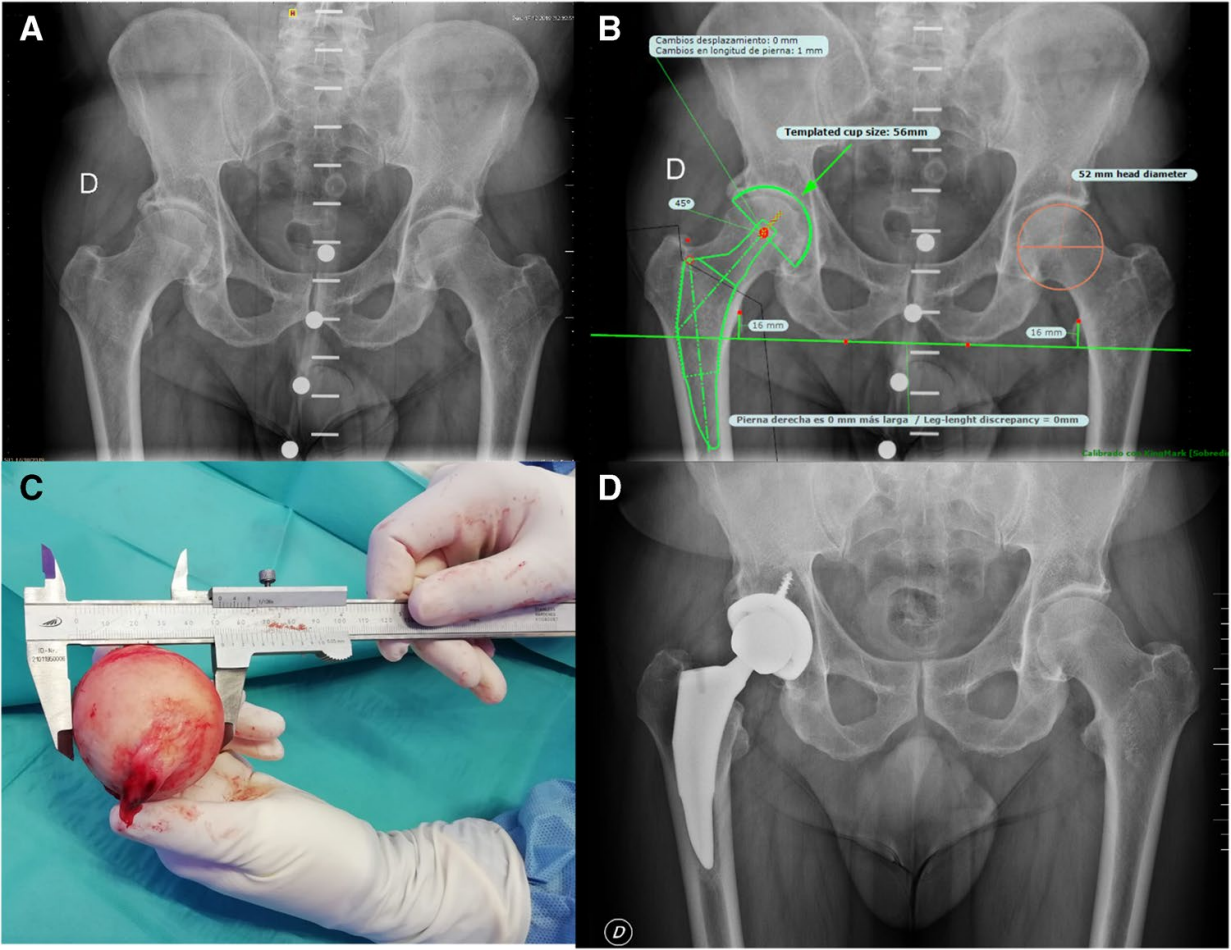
A Digital pre-operative radiograph showing the anterior–posterior projection of the pelvis with a dual calibration marker ball system (KingMark™). B Pre-operative template using the using the TraumaCad™ software in which a 56 mm diameter head was estimated. C Intra-operative measurement of the anterior–posterior diameter of the femoral head using a Vernier caliper device. In this example, a 52 mm diameter head was measured. D Post-operative radiograph after the implantation of a definitive 56 mm diameter cup
